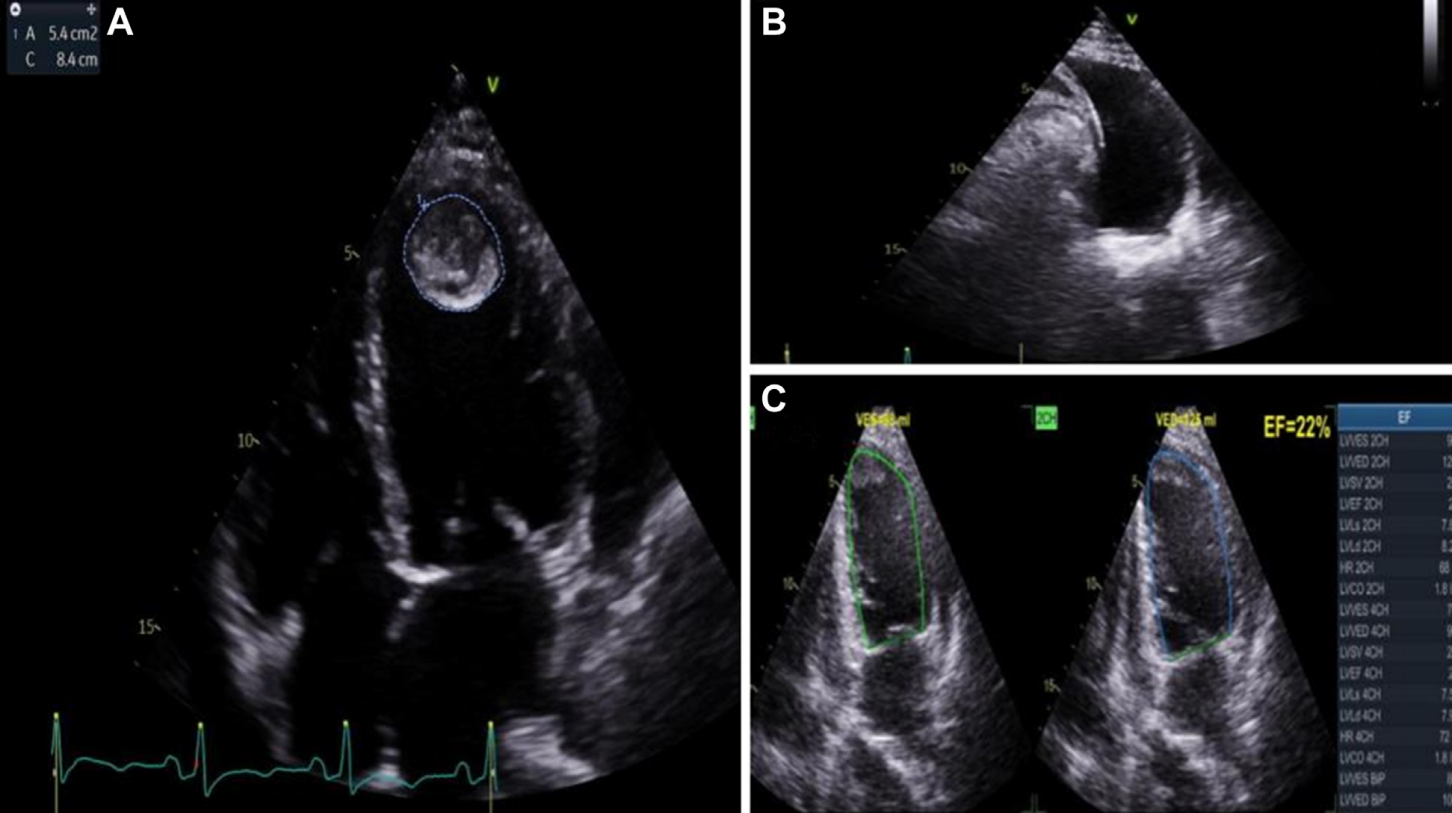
Baseline Echocardiographic Assessment at Initial Presentation (A) The 4-chamber view showed large apical left ventricular (LV) thrombus; (B) lung ultrasound revealed right-sided pleural effusion; (C) Simpson method ejection fraction (EF) measurement showed EF of 22%.
